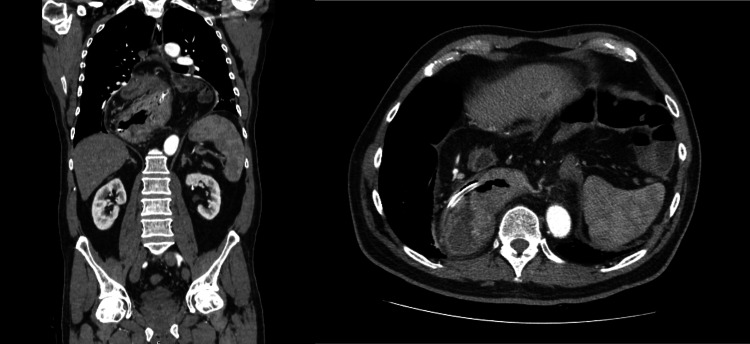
Computed tomography (coronal and transverse section) performed in the emergency department Giant HH with the pylorus positioned in a plane superior to the gastric fundus. Axial mesenteric gastric volvulus cannot be excluded.
